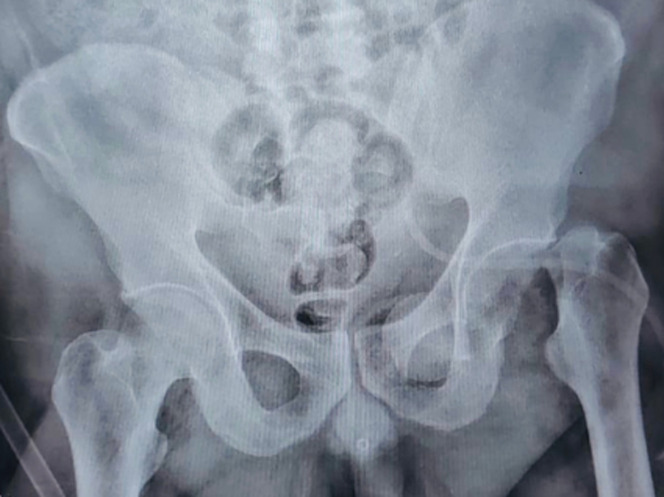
anteroposterior radiograph of the pelvis showing anterior hip dislocation and a femoral head fracture
